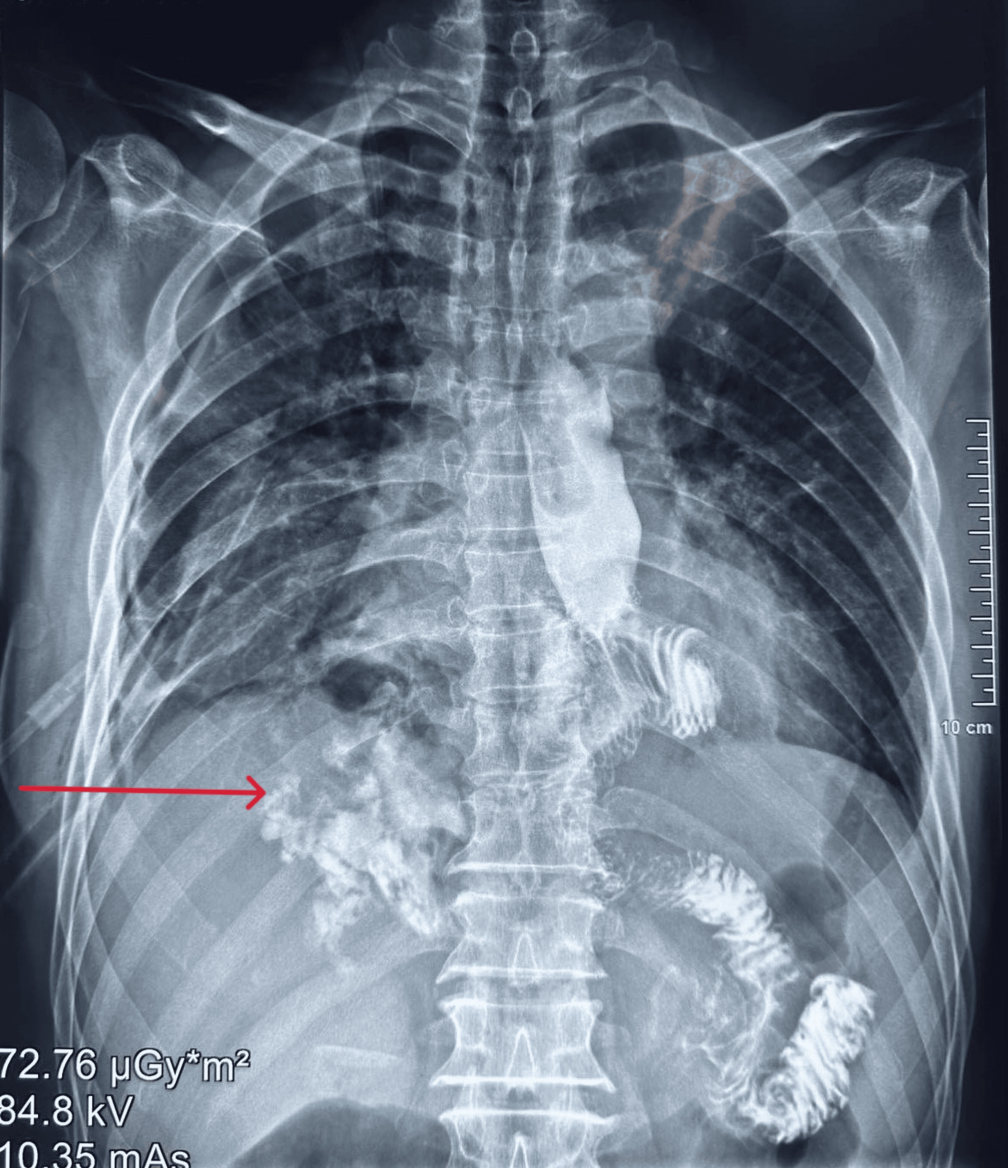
X-ray of the esophagus and stomach with contrast: note the contrast in the right chest base
